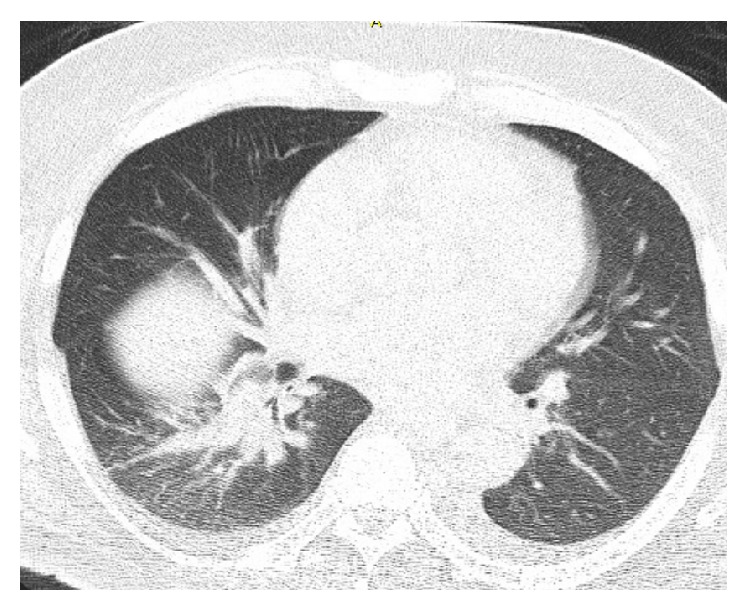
Axial image of CT of chest performed on August 17, 2013: pulmonary windows demonstrating atelectatic change or scarring in the right lower lobe.
